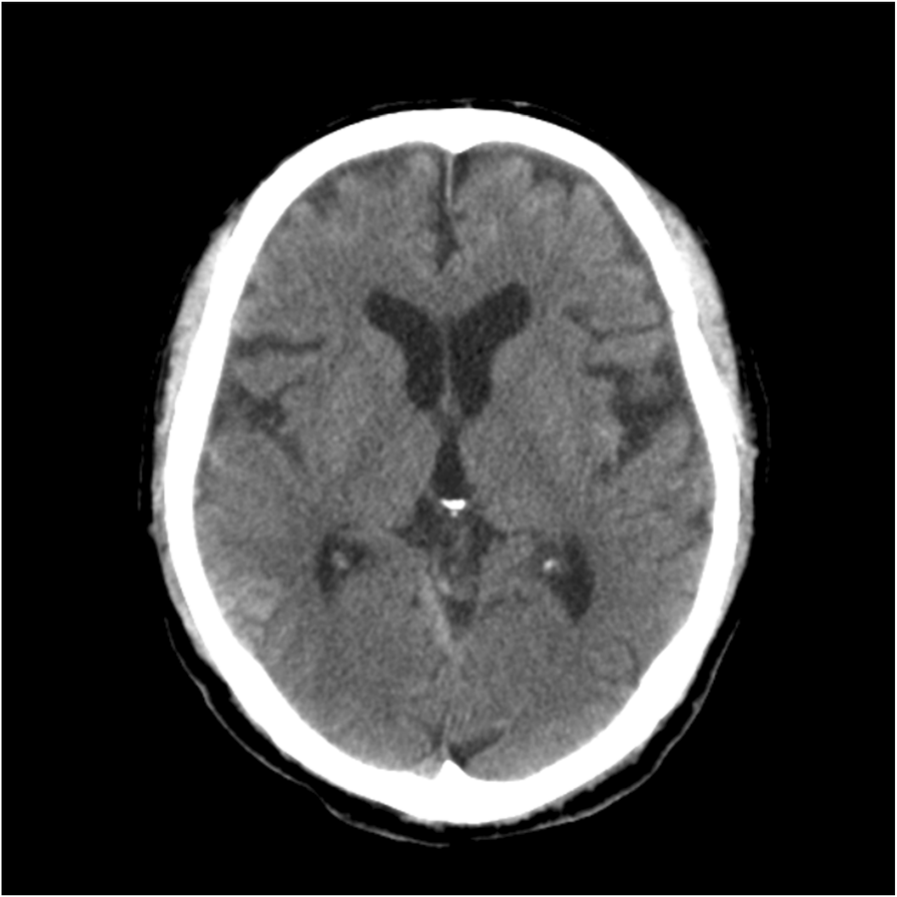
Head CT on admission showed a right temporal lobe contusion and subdural hematoma in the cerebellum tent
